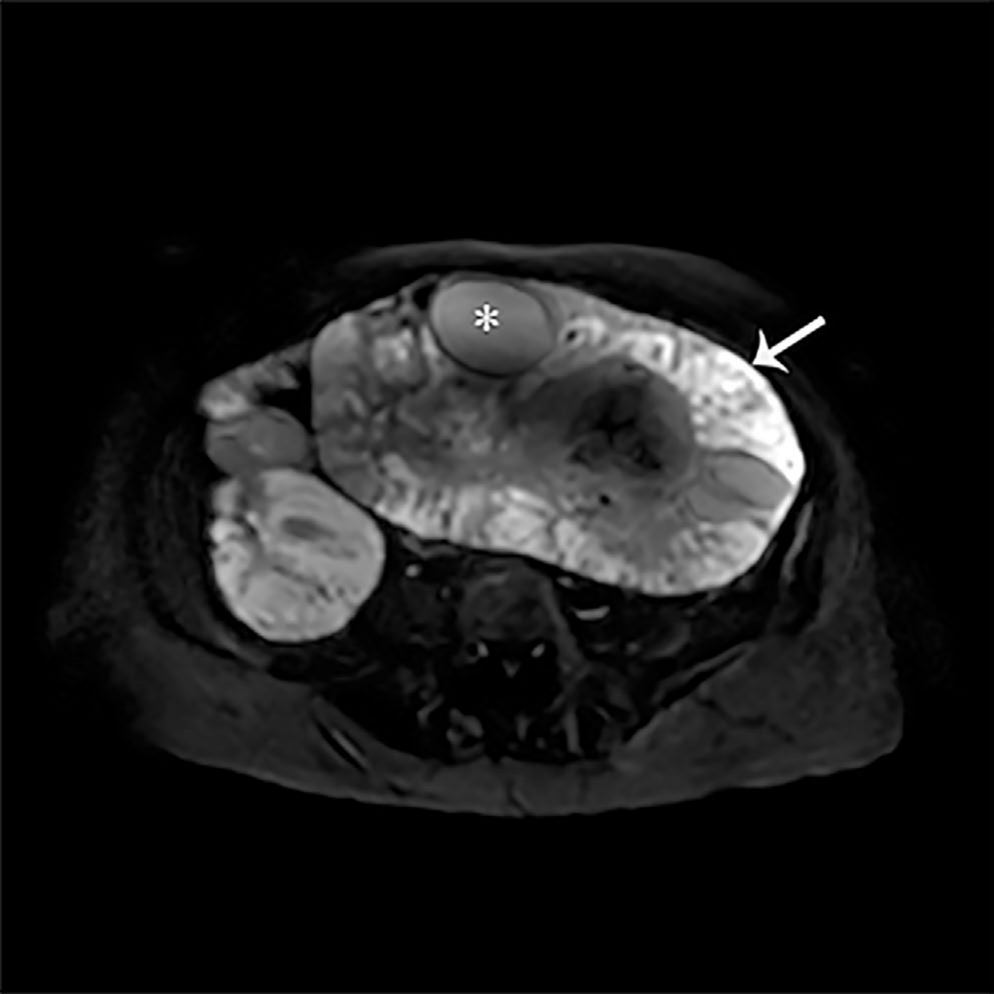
Image of MRI on diffusion‐weighted imaging (DWI), the densely populated areas of tumor cells (indicated by the white arrow) exhibit high‐signal intensity, while the cystic and necrotic regions of the tumor (indicated by *) show low signal intensity.
